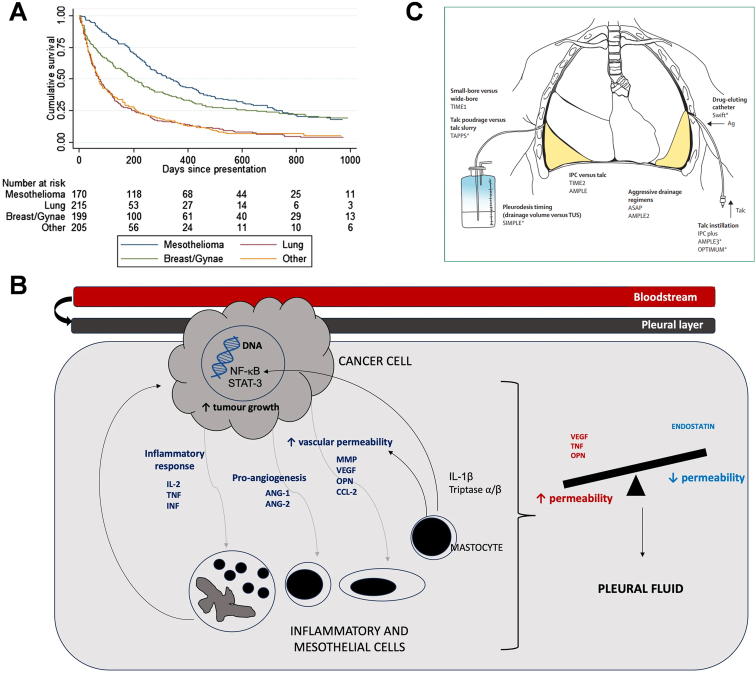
A. Kaplan–Meier survival curves according to cell type for the UK, Australian and Dutch cohorts combined [205]. Copyright 2014, BMJ B. MPE pathogenetic mechanism [206]. Copyright 2024, Respiratory Research. C. Recruited and recruiting trials in management of malignant pleural effusion Ag = silver. TUS = thoracic ultrasound. *Recruiting trials [207]. Copyright 2020, Elsevier.
